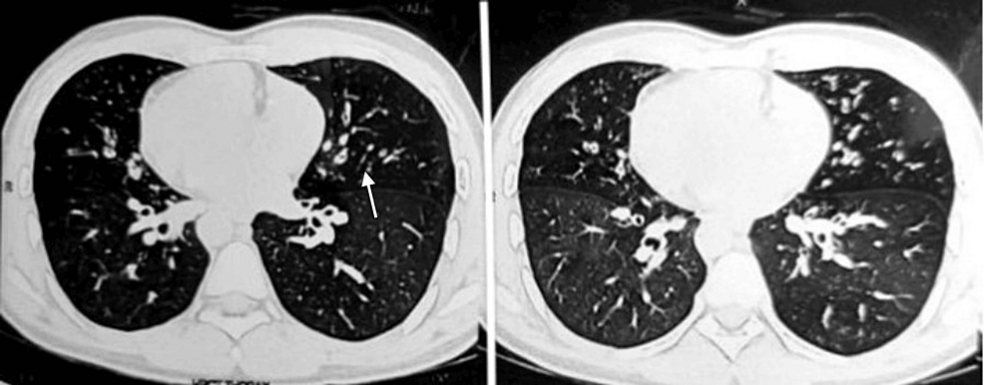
HRCT High-resolution computed tomography (HRCT) scan of the chest, revealing multiple cavitary lesions having a "signet ring" appearance and a "tram-track" sign due to bronchial wall thickening suggestive of bronchiectasis.
